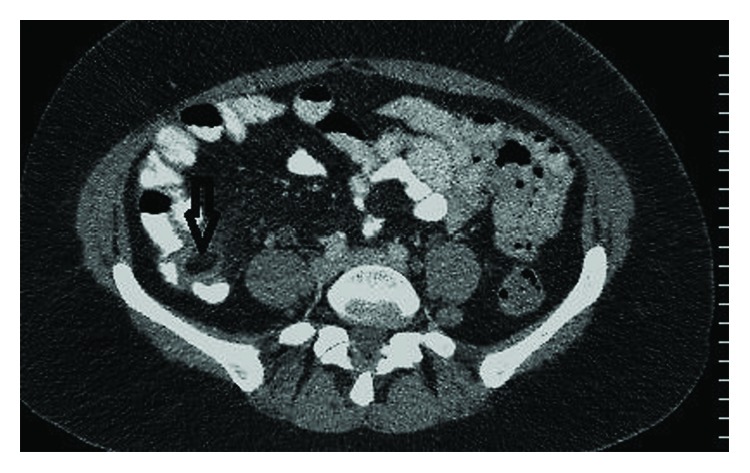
Axial CT abdomen with oral contrast showing caecal epiploic appendagitis with minor pericaecal fat stranding (vertical white arrow).
